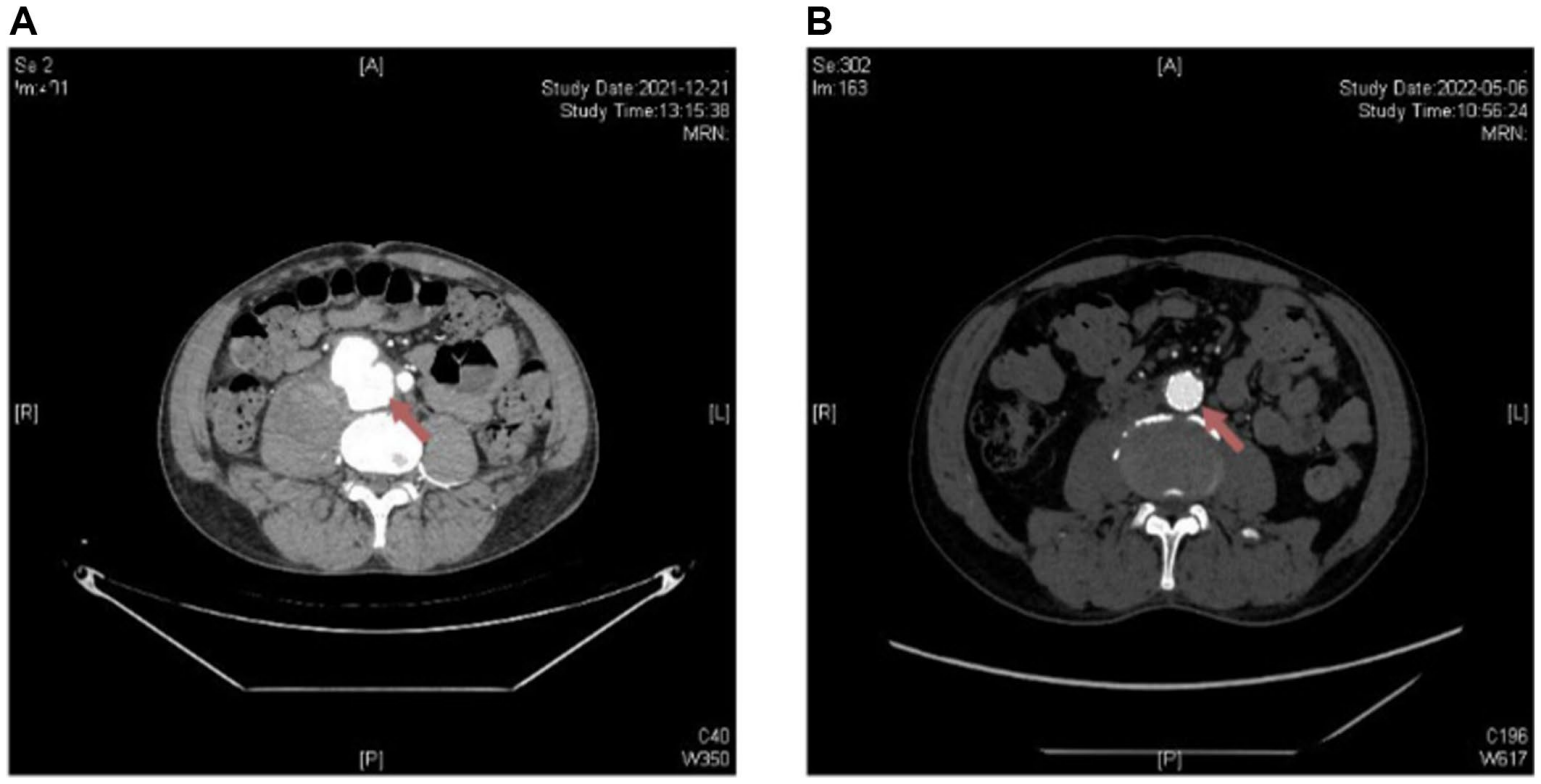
Radiological changes on abdominal aortic computed tomography angiography (CTA) were observed in Patient 1 before and after treatment. (A) Upon admission: the density of the abdominal aorta and right iliac artery is uneven, with wall thickening and roughness, and the fat planes appear indistinct. (B) After treatment: the walls of the abdominal aorta and right iliac artery show slight thickening, with significant improvement compared to the previous examination.
